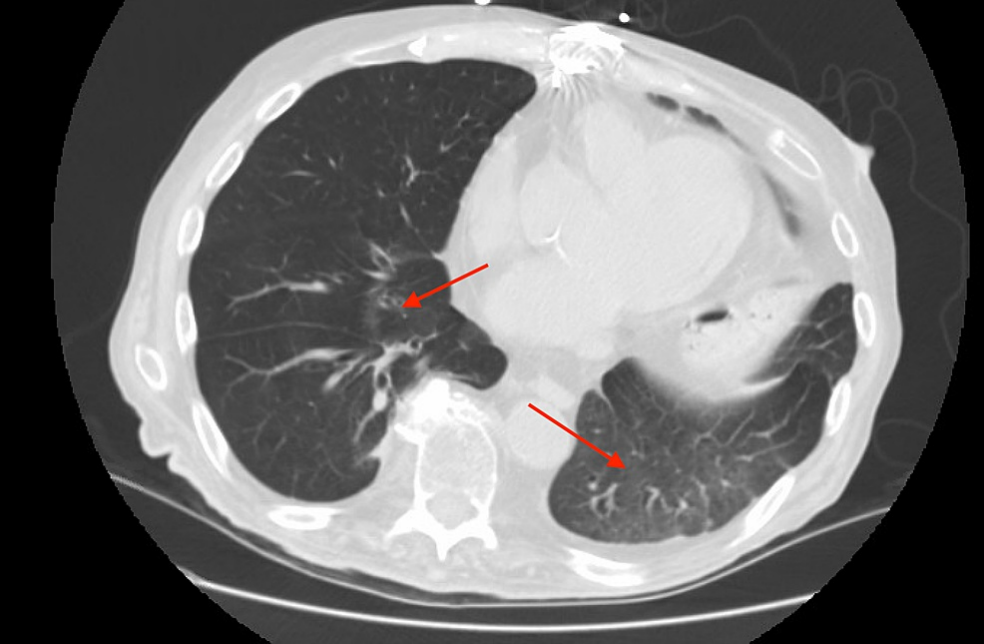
Repeat chest CT after three months of treatment showing improvement of infiltrates (arrows)
